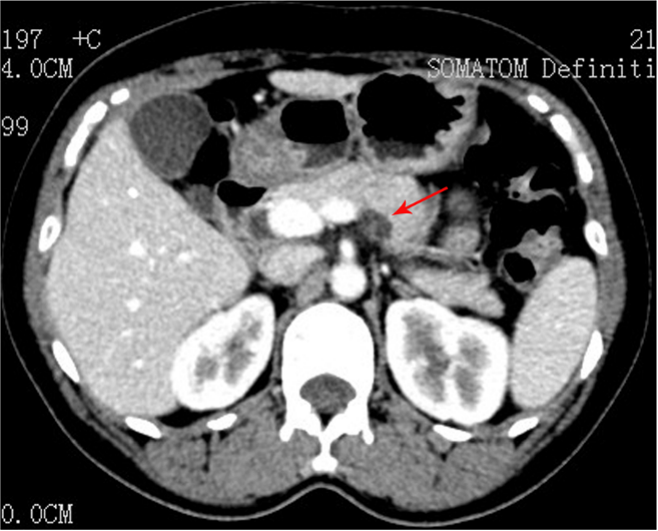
Computed tomography scan showing a 10 × 14-mm cystic neoplasm (arrow) in the proximal body of the pancreas.
